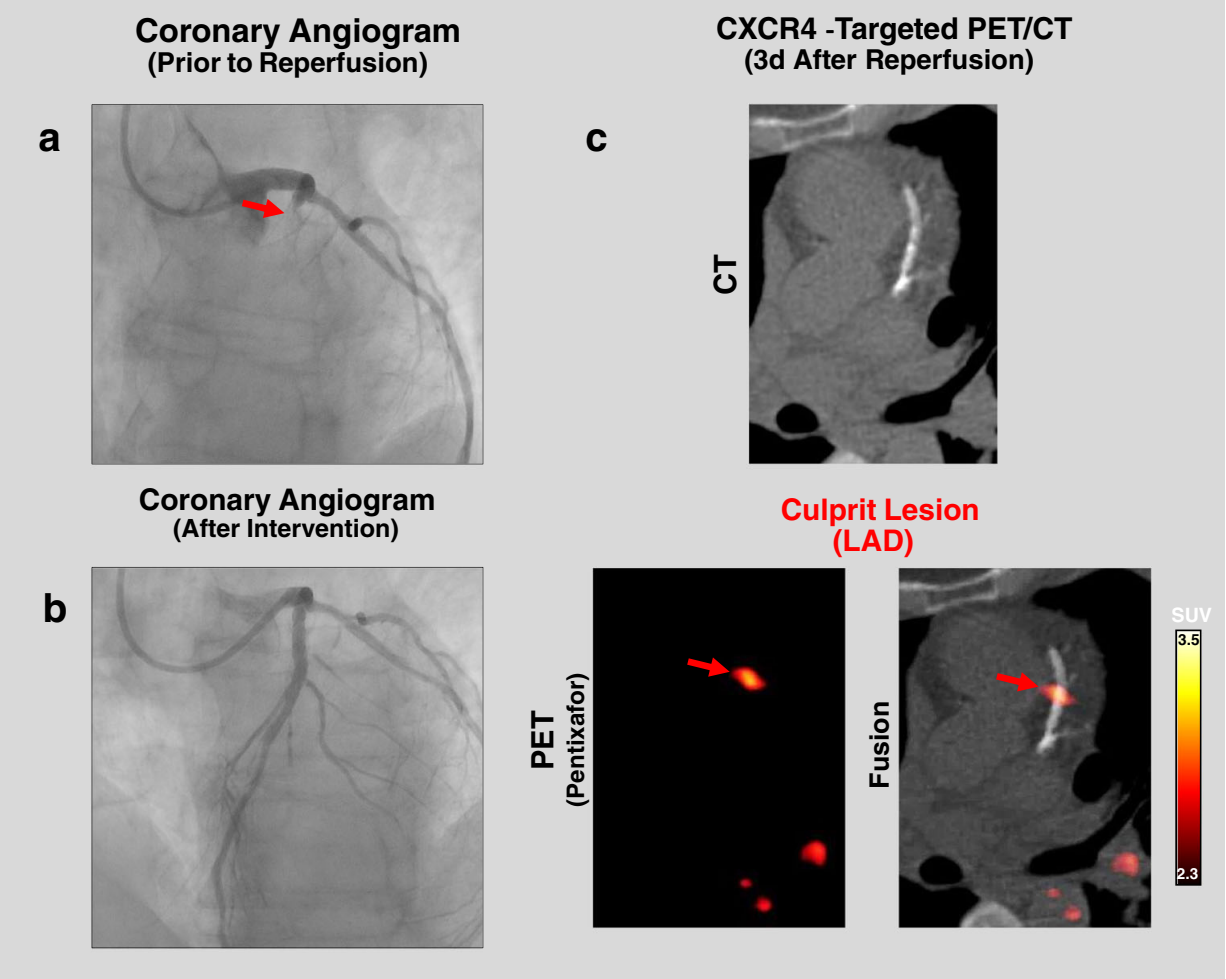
[^68^Ga]Pentixafor PET/CT identifies CXCR4 upregulation in culprit coronary lesions after acute myocardial infarction and stent-based reperfusion. a Coronary angiogram prior to reperfusion shows LAD occlusion (*arrow* lesion). b Coronary angiogram after stent-based LAD reperfusion. c [^68^Ga]Pentixafor PET/CT after reperfusion showing focal CXCR4 signal at the site of the LAD culprit lesion fusing to the stent location (i.e. CXCR4^+^ culprit lesion)
